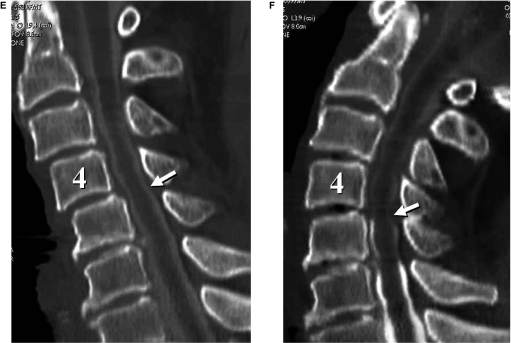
An 84-year-old man with C4/5 myelopathy. A, B, and C: Plain X-ray (A: flexion position, B: neutral position, and C: extension position). In the flexion position, 2-mm anterior slippage in C3 and 3-mm anterior slippage in C4 are demonstrated (A). In the extension position, C3 anterior slippage is reduced, and C4 anterior slippage is 1 mm (C). Space available for the spinal cord at C4/5 in the flexion and extension positions is 12 and 15 mm, respectively. C5-7 range of motion and lordosis angles are 3° and 4°, respectively. D: MRI showed spinal cord compression at C3/4 and C4/5. E and F: CT after myelography (E: flexion position and F: extension position). In the flexion position with maximum anterior slippage and osseous stenosis, deformation due to spinal cord compression is not confirmed (E). In the extension position with minimum anterior slippage and osseous stenosis, findings indicative of spinal cord compression from the posterior direction due to the buckling of the ligamentum flavum are confirmed (F).
